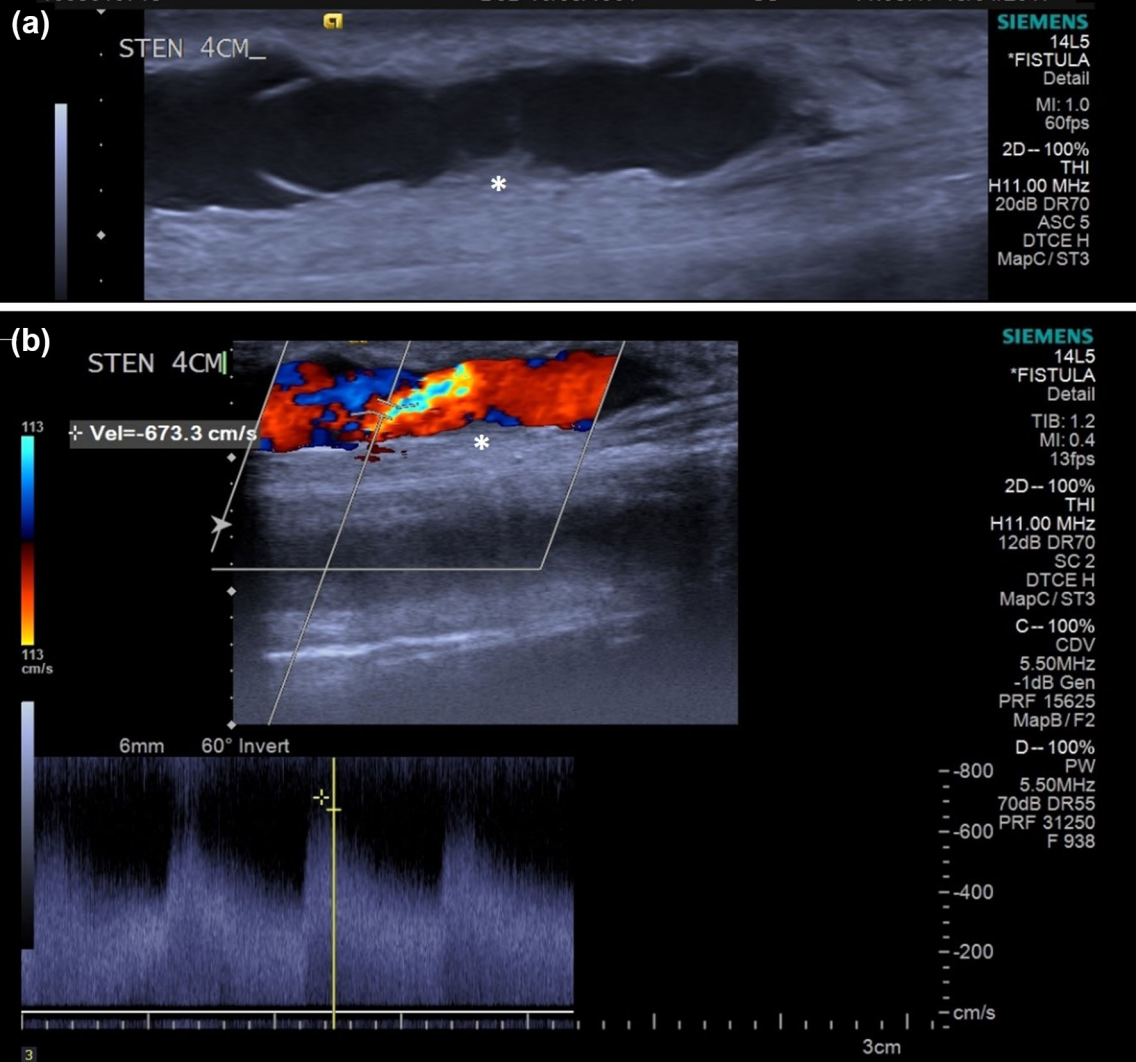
(a) US b-mode image of the vein-side of an AVF with a stenosis (marked with an asterisk). Also visible are the leaflets of a venous valve fixed in position. (b) US b-mode image of the same area superimposed with the colour Doppler data, and with PSV recorded at 6.733 m/s at the location within the crosshairs (stenosis again marked with an asterisk).
